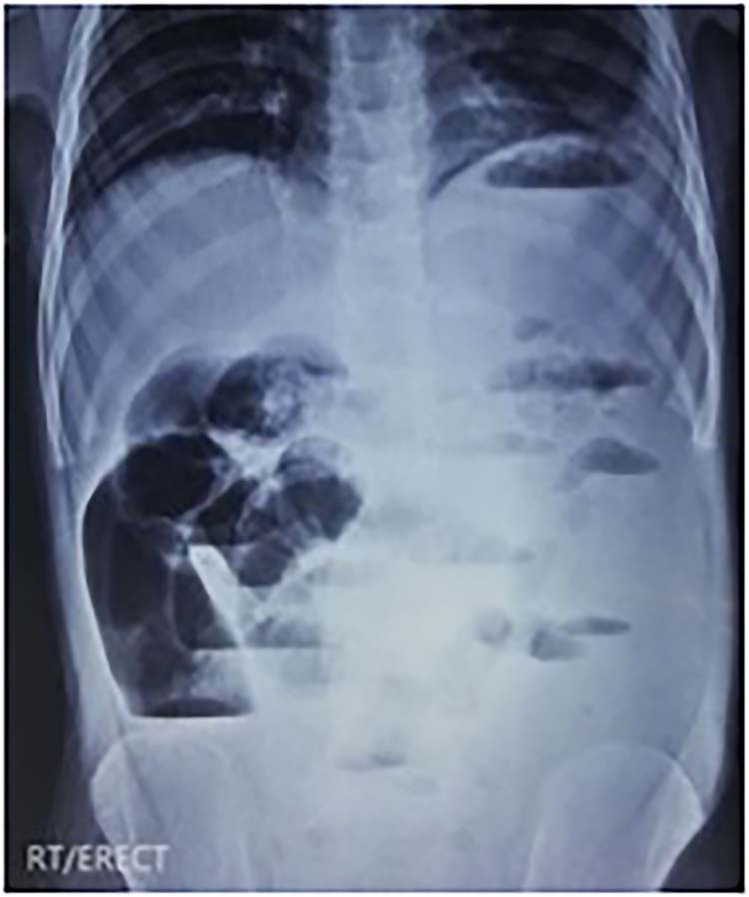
X-ray of abdomen erect view showing multiple air-fluid levels.
